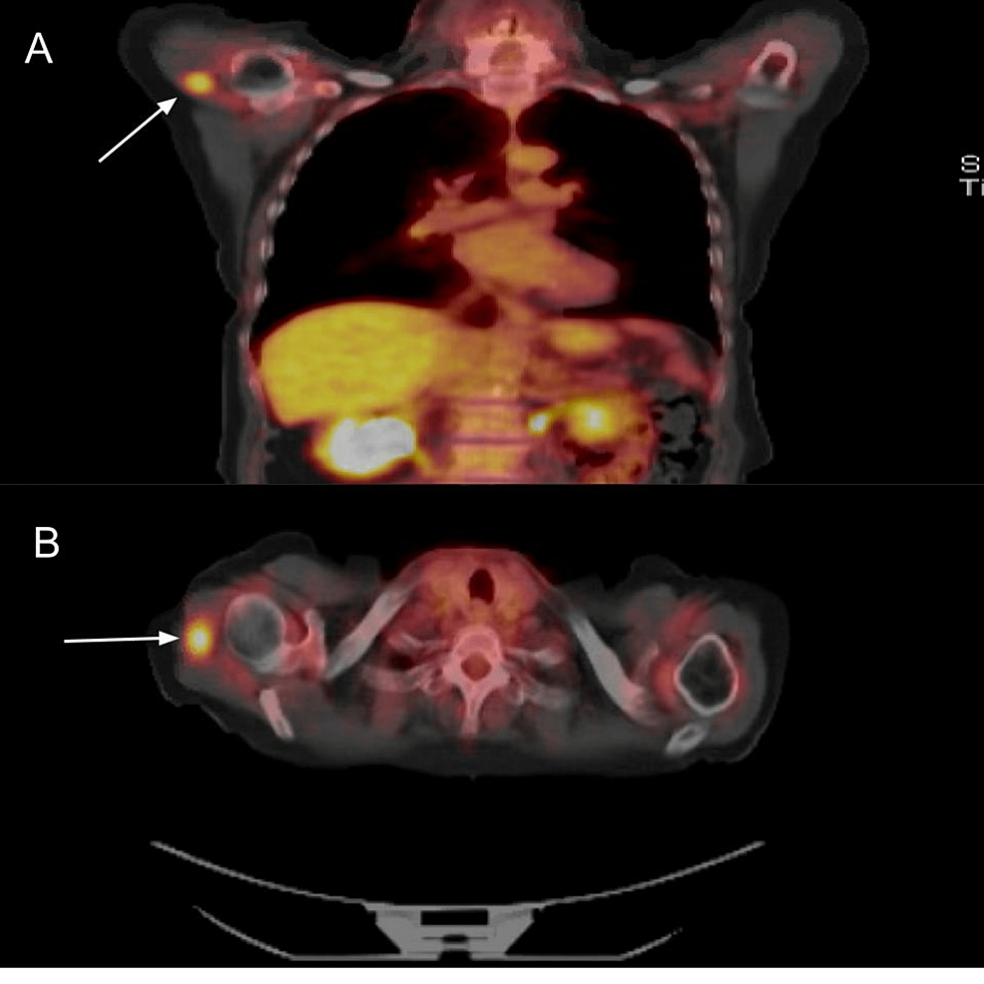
PET-CT showed an abnormal focal area of increased activity (white arrow) localizing to the proximal right deltoid muscle (A: coronal view, B: axial view). PET-CT: Positron emission tomography-computed tomography.
